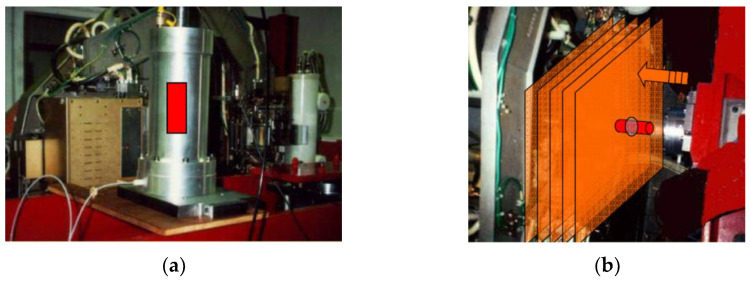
Tomotriax: (a) triaxial cell with selected sample, (b) triaxial cell placed in CT ready for operation in the scanner; (source: photo of Desrues J. from 3S-R Grenoble and LMA Marseille, 2000; after [109], available via 2021 Desrues’ permission).
